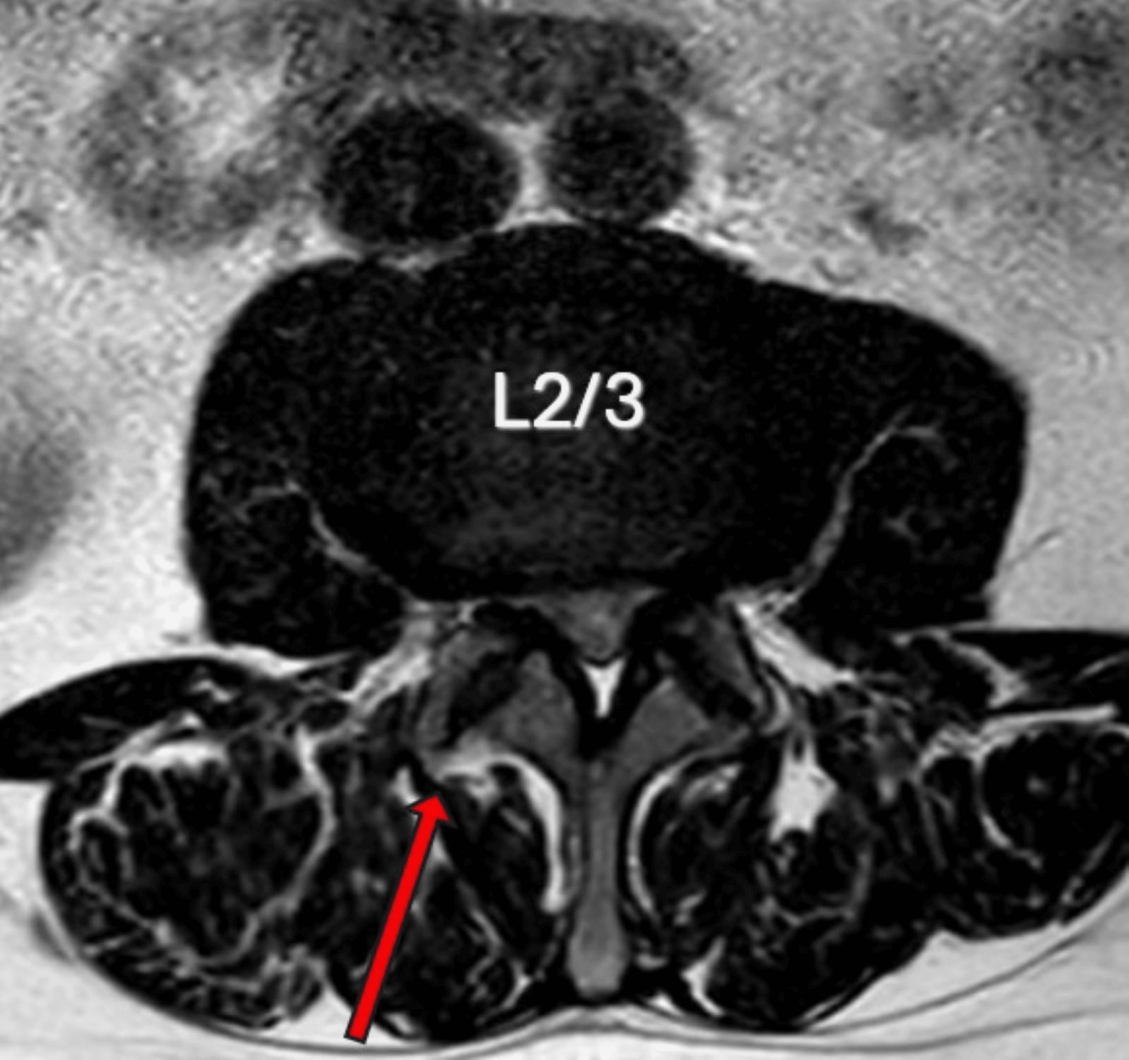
Axial T2 MRI of the lumbar spine (L2/3) demonstrating significant degenerative lumbar stenosis secondary to bilateral facet hypertrophy (R > L) with significant compression of the lateral recesses bilaterally (R > L). The red arrow demonstrates the right-sided approach used for the minimally invasive unilateral laminotomy and bilateral decompression and also highlights the side of the foot drop. R: right; L: left
